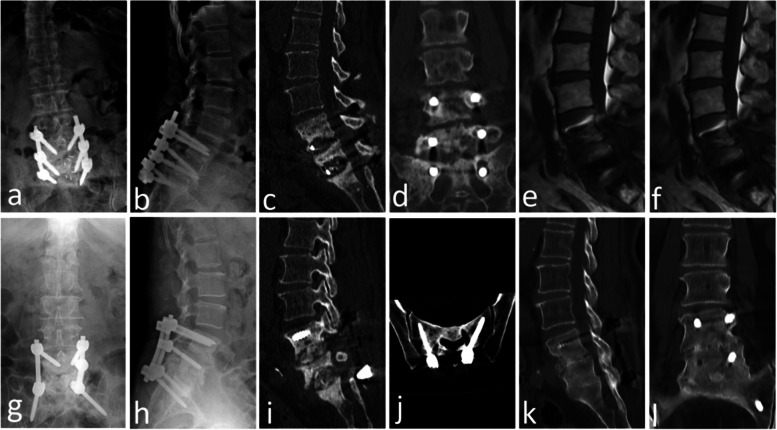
Case No. 1. A 63-year-old female underwent orthopedic revision surgery of lumbosacral fusion due to the complication of L4/5 and L5/S1 lumbosacral chronic pyogenic spondylodiscitis. S1 alar screws were used as substitutes for S1 pedicle screws to enhance biomechanical stability. (a–f) Preoperative X-ray, CT and MRI scans showed bone destruction with significant vertebral body loss at L4, L5 and S1. (g, h) X-ray images 3 days after the operation showed autologous grafting, and screws and rods were in good locations. (i–j) CT images 1 month after surgery showed the implanted bones and the trajectory of S1 alar screws in axial view. (k–l) CT images 19 months after surgery showed solid bone fusion from L4 and S1 without screws loosening
